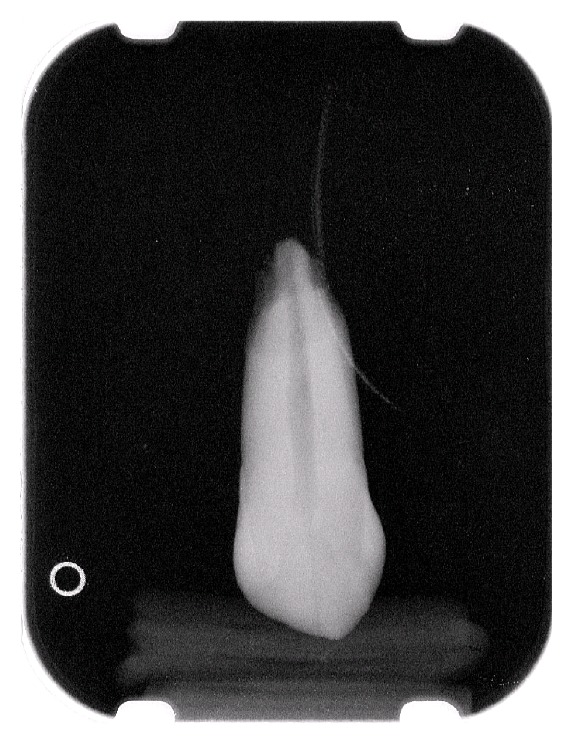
Digital endooral X-ray: rizalysis.
